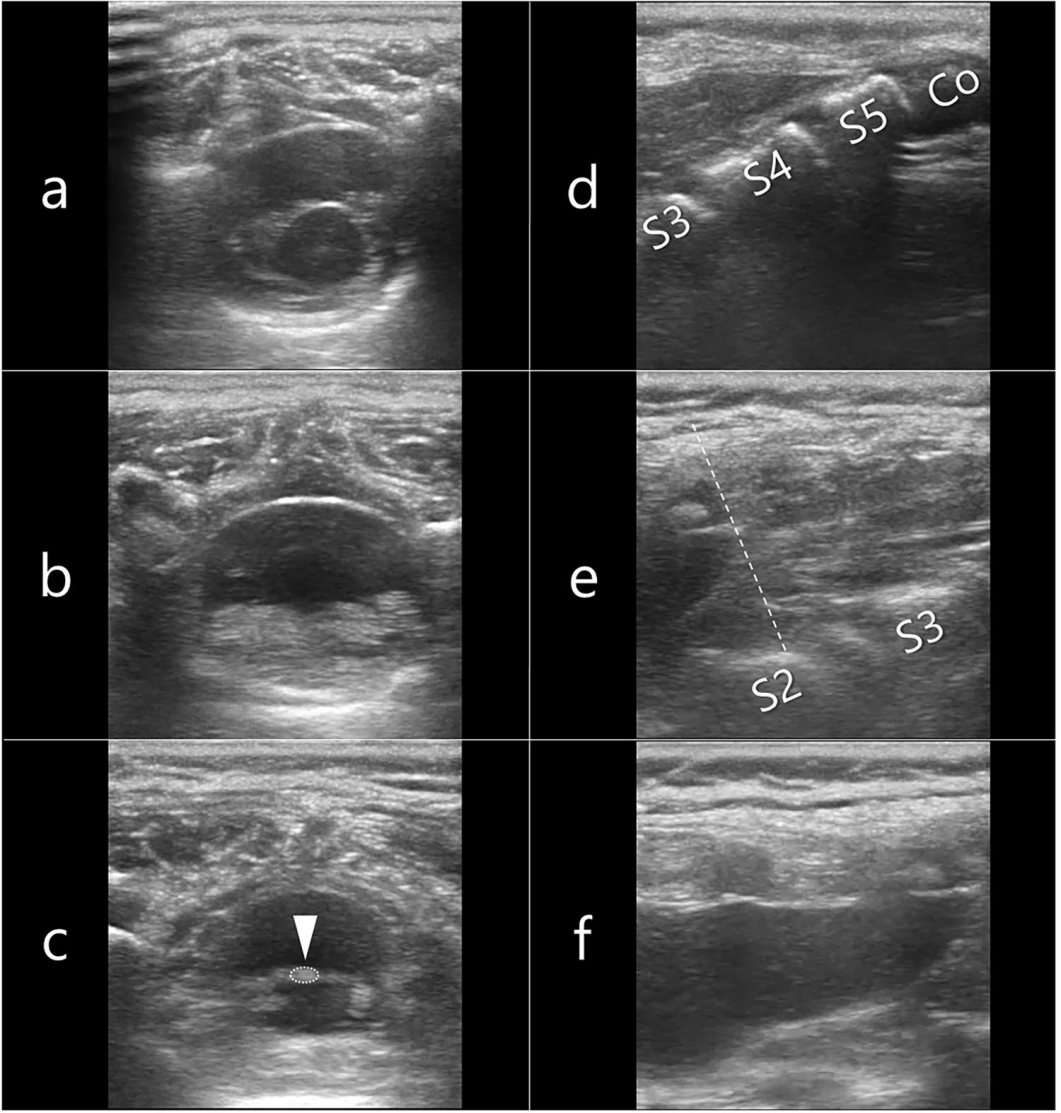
Key imaging findings from systematic spinal ultrasound screenings of a 16-month-old child. a Horizontal image between T12 and L1, showing the conus medullaris and cauda equina. b Horizontal image between L2 and L3, predominantly displaying the cauda equina within the dural sac, as the conus medullaris typically terminates at the L2 level. c Horizontal image between L5 and S1, where the filum terminale (arrow) can be identified due to dispersion of the cauda equina. d Sagittal image on which the sacral level can be determined by the position of the coccyx. e Sagittal image on which the structure in the sacral canal can be assessed, and the end of the dural sac (dotted line) can be identified. f Sagittal image showing structures within the dural sac, including the filum terminale. *Co* coccyx, *S* sacrum
